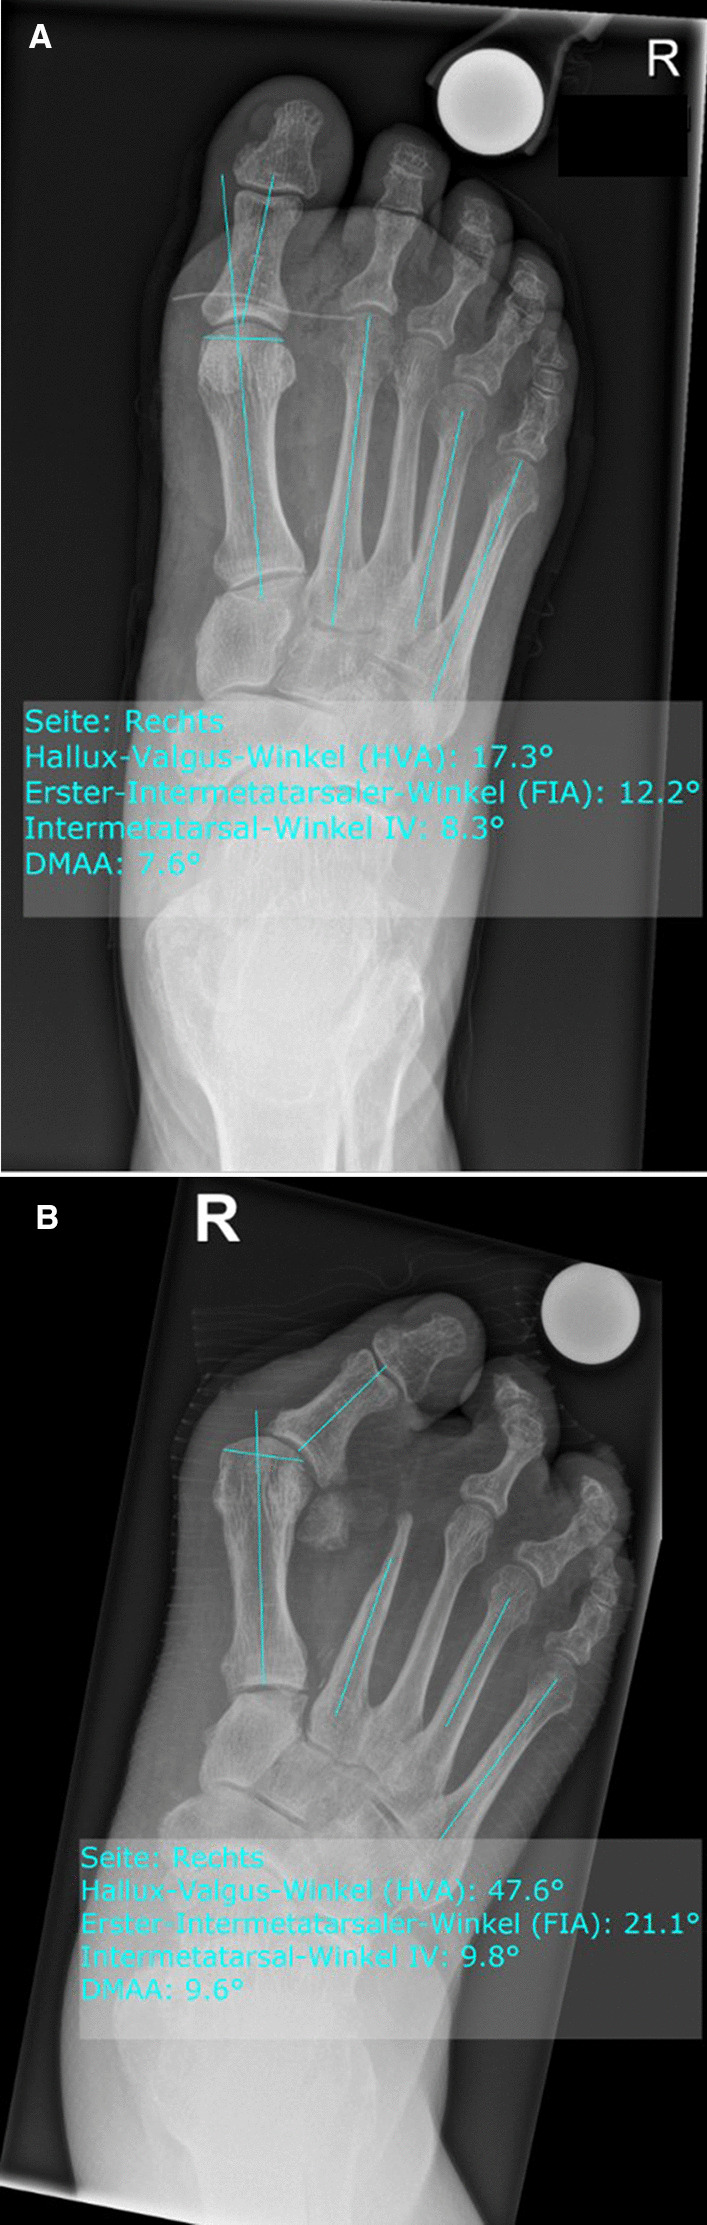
A. Preoperative anteroposterior right forefoot radiograph of a 60 years old male patient with marked first metatarsophalangeal angle (HVA), first–second intermetatarsal angle (IMA, here IV) and distal metatarsal articular angle (DMAA). B. Postoperative anteroposterior forefoot radiograph of the same patient 5 years after transmetatarsal amputation of the second ray with marked HVA, IMA and DMAA
